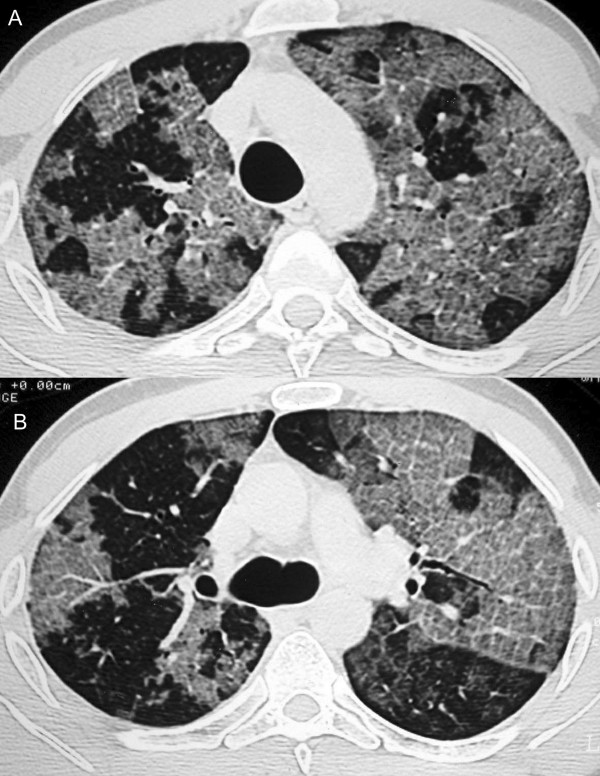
A and B: High resolution CT of the lungs demonstrates diffuse ground-glass attenuation with superimposed interlobular septal thickening ("crazy-paving" pattern), mainly in the superior lobes.
